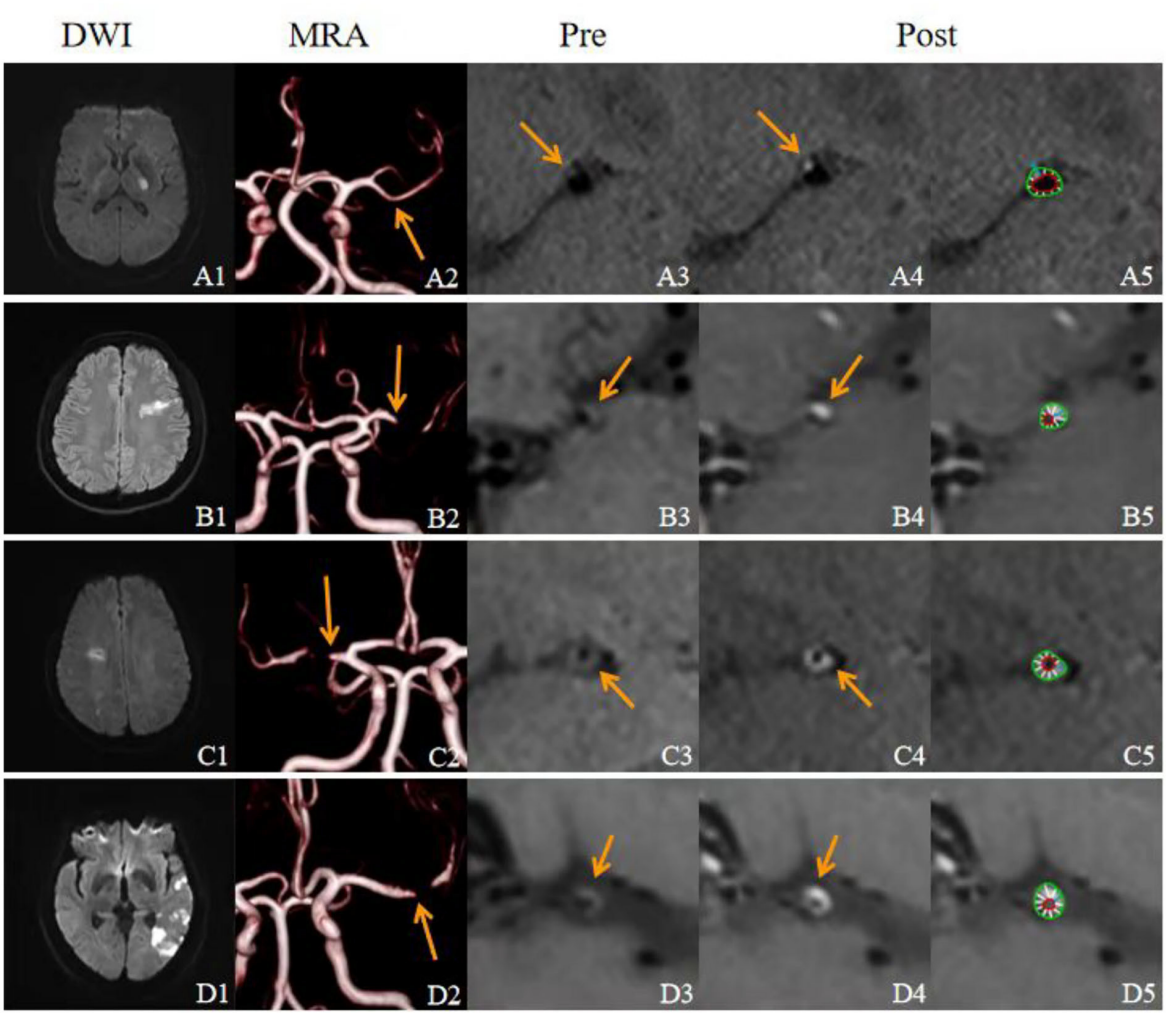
Characteristics of the culprit plaque in the M1 segment of the MCA in four patients with acute stroke with different mechanisms. (A1–A5), A 39-year-old male, diffusion-weighted images (DWIs) (A1) showed high signal intensity in the left basal ganglia, and the stroke mechanism was parent artery atherosclerosis occluding penetrating artery by MCA atherosclerosis. Magnetic resonance angiography MRA_ (A2) showed that the left MCA M1 lumen was normal (arrow). Sagittal images of the precontrast (A3) and postcontrast (A4) HR-VWI showed that the plaque was located in the upper wall (arrow), showed mild enhancement, and the inner and outer walls of vessels were semiautomatically delineated on postcontrast HR-VWI (A5). (B1–B5) A 48-year-old male, DWI (B1) showed multiple hypersignals in the left frontal cortex and the subcortical area, and an artery-to-artery embolism was considered the mechanism of stroke; MRA (B2) showed that the left MCA M1 lumen was severely stenotic (arrow); (B3,B4) HR-VWI showed that the plaque was located in the upper wall, which showed obvious enhancement (arrow). (C1–C5), A 53-year-old male, DWI (C1) showed multiple hypersignals in the right subcortical watershed, and the mechanism of stroke was considered when evaluating the mechanism of hypoperfusion. MRA (C2) showed that the right MCA M1 lumen was severely stenotic (arrow). (C3,C4) HR-VWI showed that the plaque was located in the posterior wall, which showed obvious enhancement (arrow). (D1–D5), A 60-year-old male, DWI (D1) showed multiple hypersignals in the left temporal lobe and the left posterior cortical watershed area; MRA (D2) showed that the left MCA M1 lumen was severely stenotic (arrow); (D3,D4) HR-VWI showed that the plaque was located in the posterior wall, which showed obvious enhancement (arrow).
